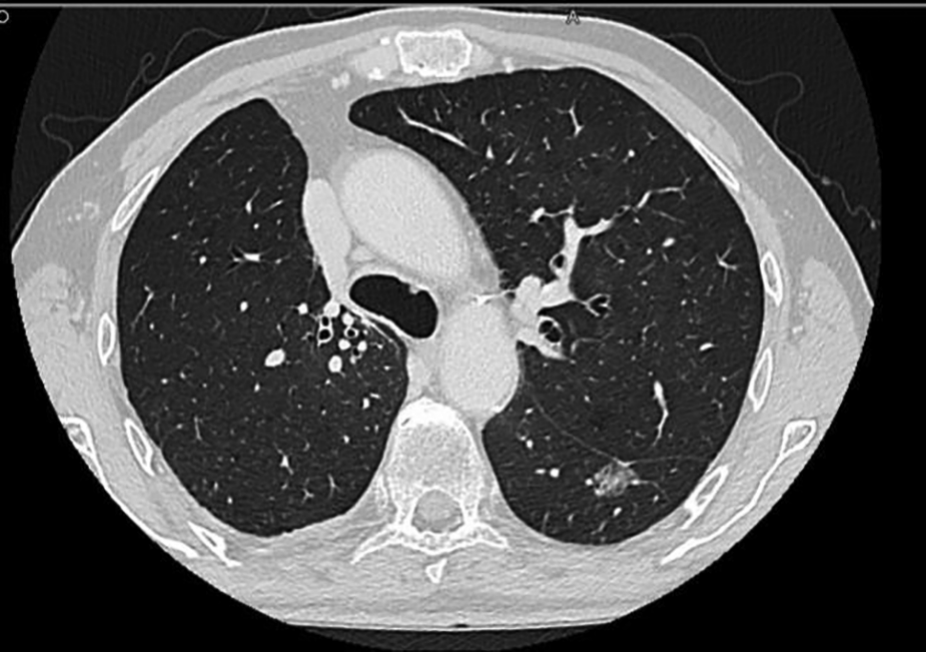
Pre-operative CT-scan of the left S6 segmentectomy showing the lesion.
